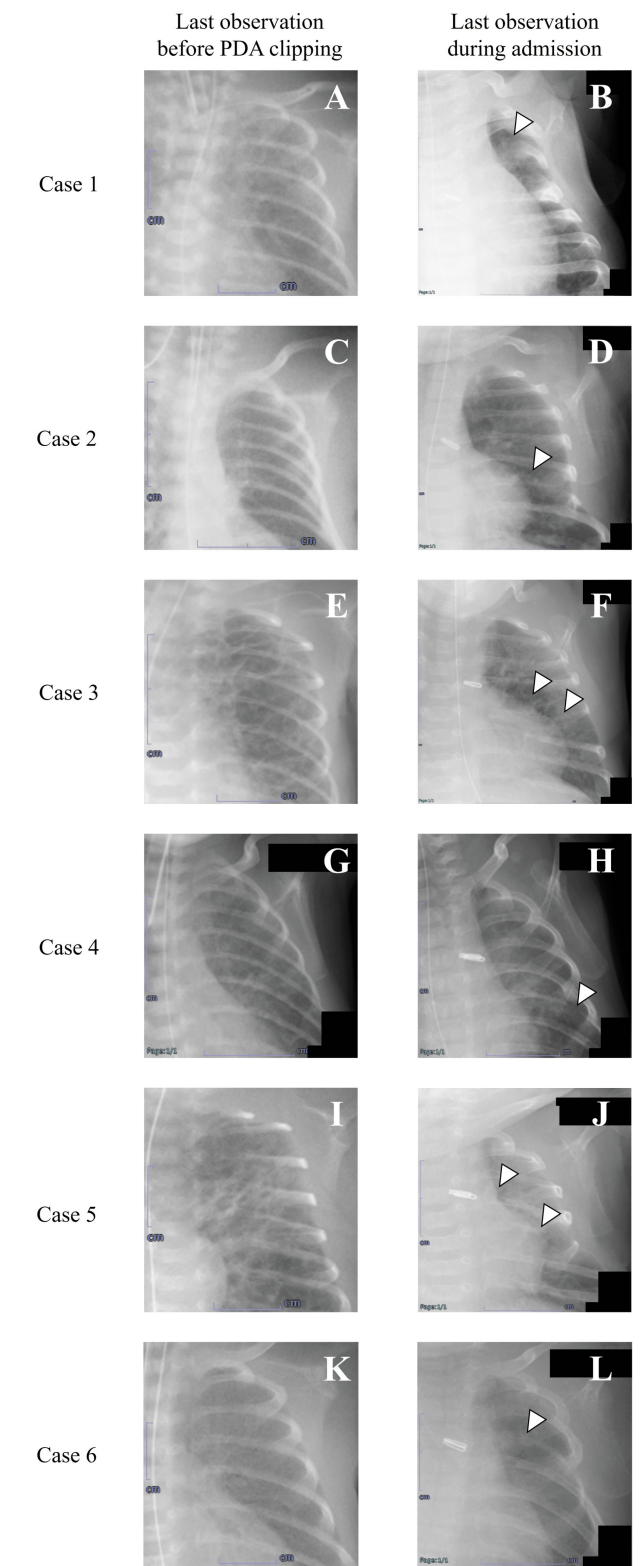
Serial plain chest radiographs demonstrating the temporal evolution of structural rib deformities in six preterm infants during hospitalization. White arrowheads indicate sites of radiographically evident rib modifications. Case 1 (panels A and B) shows disappearance of the third rib; Case 2 (panels C and D) shows narrowing of the sixth rib; Case 3 (panels E and F) shows narrowing of the fifth rib and disappearance of the sixth rib; Case 4 (panels G and H) shows disappearance of the sixth rib; Case 5 (panels I and J) shows disappearance of the fourth rib and narrowing of the fifth rib; Case 6 (panels K and L) shows disappearance of the fourth rib. PDA, patent ductus arteriosus.
